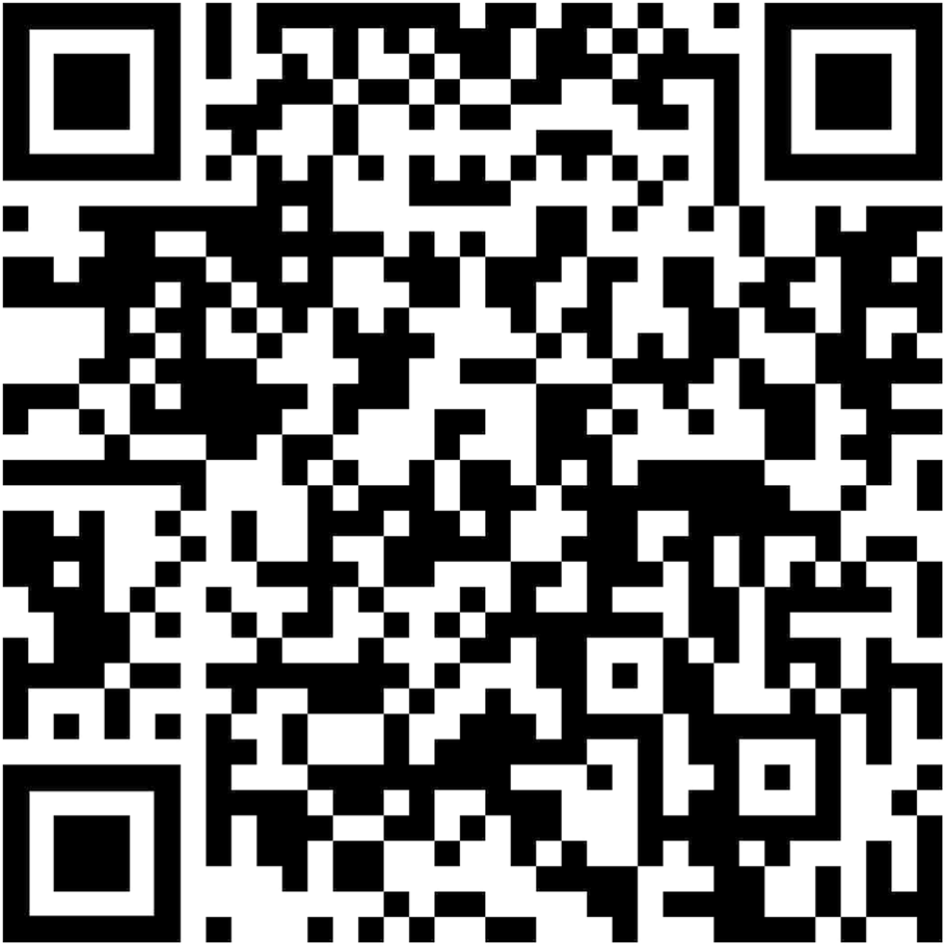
QR code to access a link to the CT acquisition protocol survey website by scanning the code
